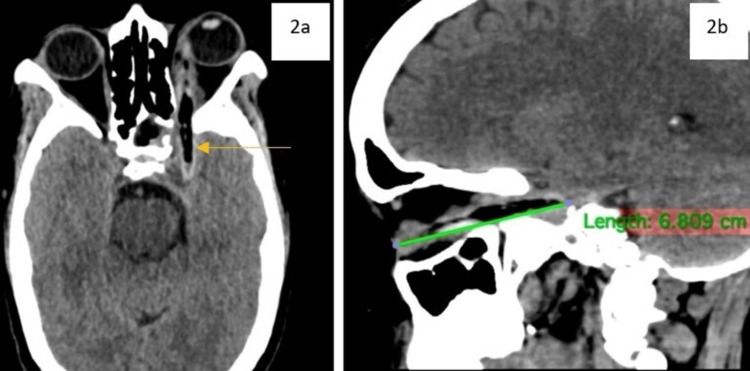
CT images. a and b: non-contrast computed tomography axial and sagittal view showing a linear hypodense foreign body of size 6.8 cm x 4.7 mm extending from the orbit to the left cavernous sinus passing through the superior orbital fissure.
